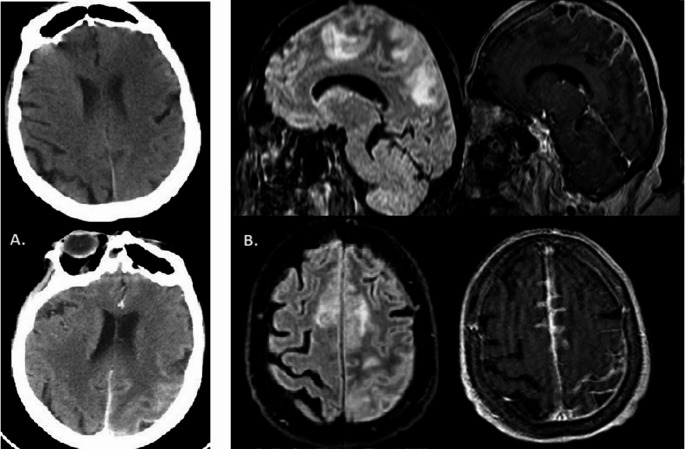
(A) Brain CT pre and post contrast. A hypodense area at the left corona radiata parietally. There is a slight enhancement following the cortex. (B) Brain MRI, FLAIR and T1-WI post contrast sequences, parasagittal and axial planes: There is increased signal intensity in the subcortical white matter in the left parietal lobe and superior frontal gyrus bilaterally. The cortex looks slightly swollen in the left superior parietal lobule.Enhancement within sulci of the left parietal and in a smaller extent to the interior surface of superior frontal gyrus
